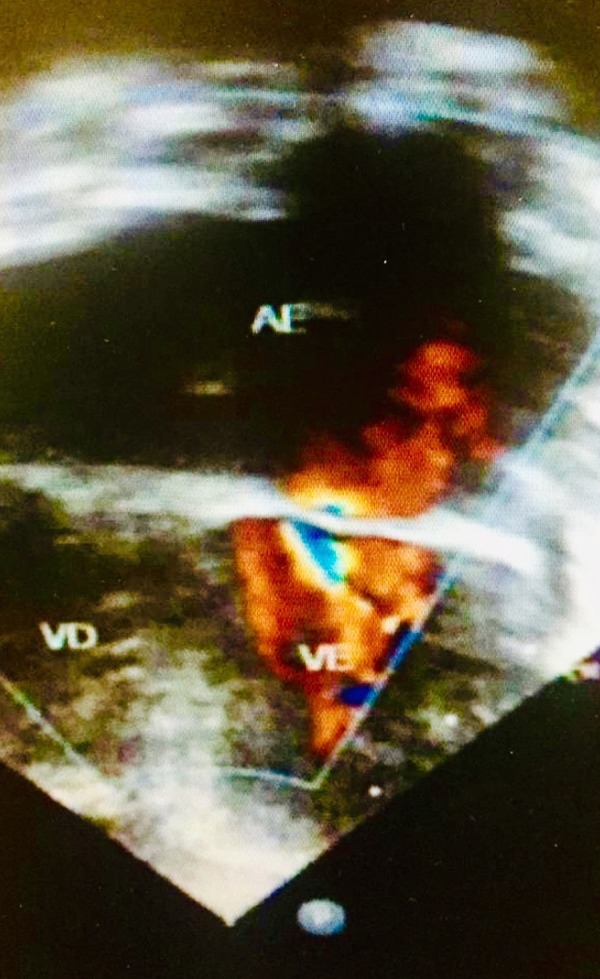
Two-dimensional echocardiography showing supravalvar mitral ring 225 x 400mm. AE=left atrium; VE=left ventricle; VD=right ventricle
